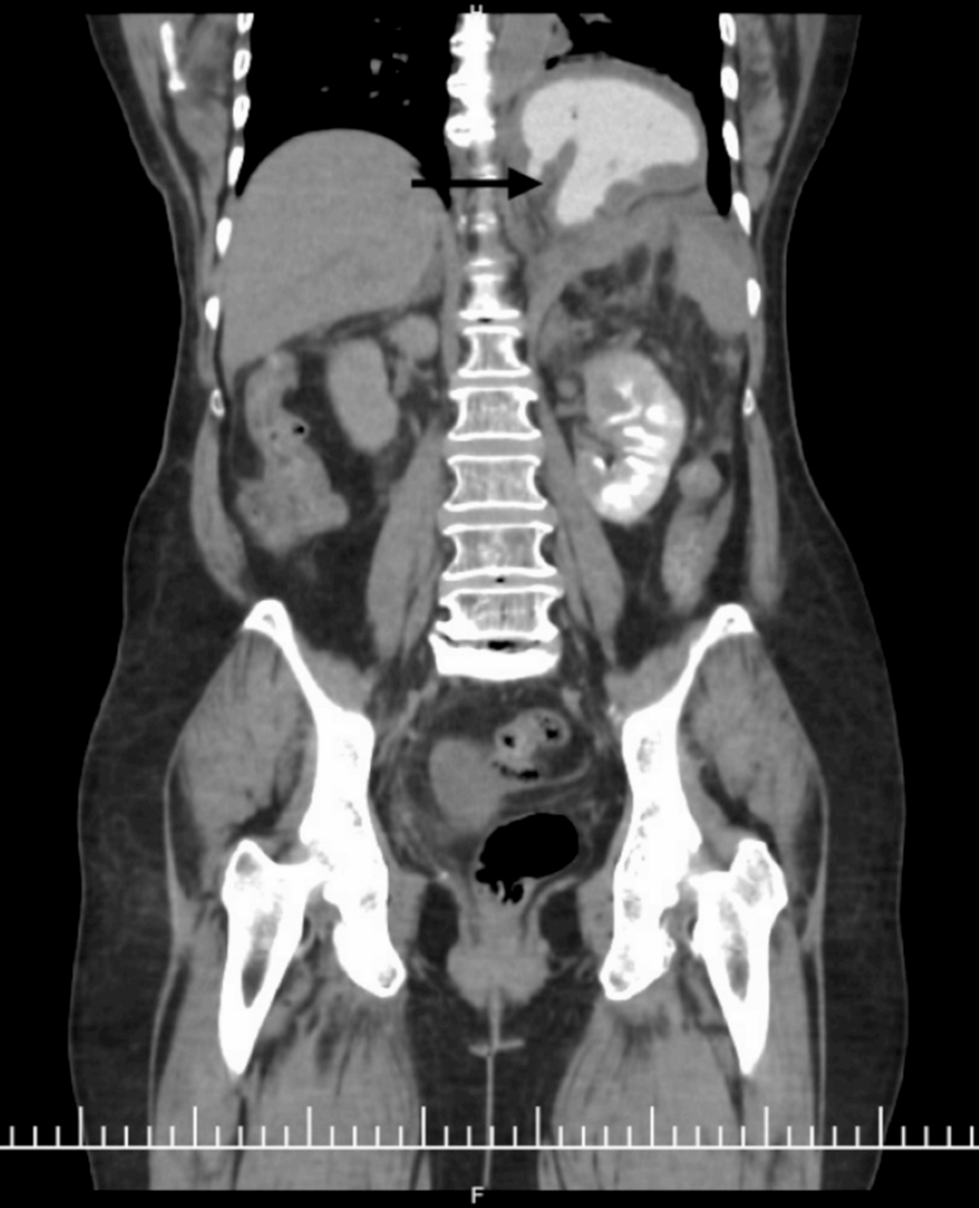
Coronal view of contrast-enhanced computed tomography (CECT) of the abdomen with the pylorus displaced superiorly above the fundus (arrow).
